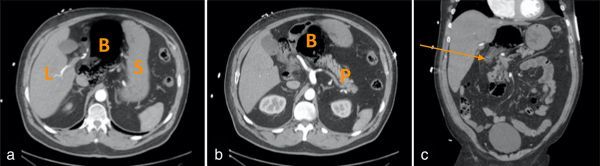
Arterial phase CT scan of the abdomen and pelvis (day 1). (a) Axial section showing hepatic flexure (B) with intraluminal faeces between the stomach (S) and liver (L), displacing the gastrohepatic ligament. (b) Axial section showing loop of hepatic flexure (B) within the lesser sac, between the pancreas (P) and the pyloric antrum. (c) Coronal section demonstrating a segment of the ascending colon passing through the epiploic foramen (arrow) into the lesser sac; the hepatic flexure is seen superior to the pancreas and medial to the stomach.
